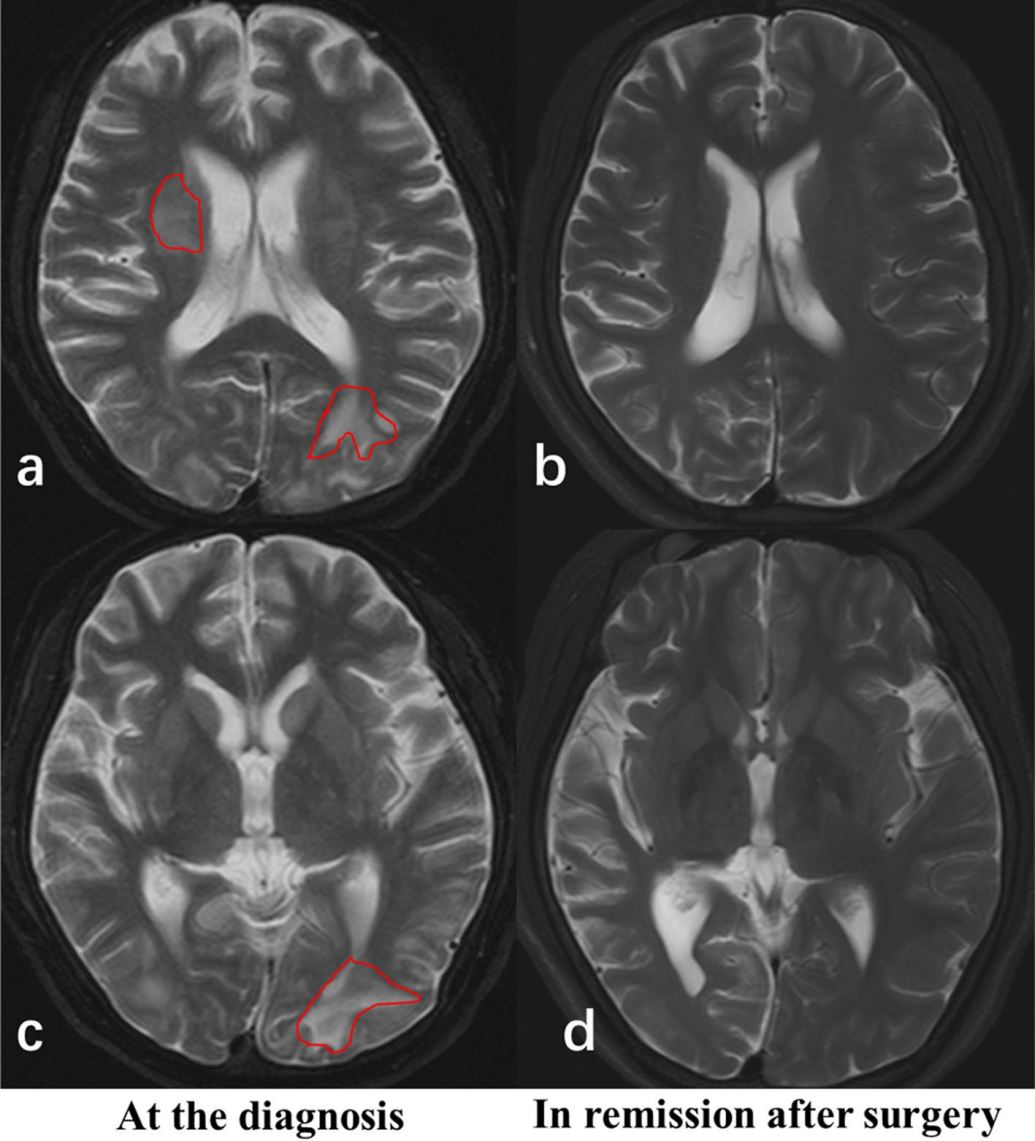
MRI image of a 19-year-old CD patient at the diagnosis (a, c) and in remission after surgery (b, d).
